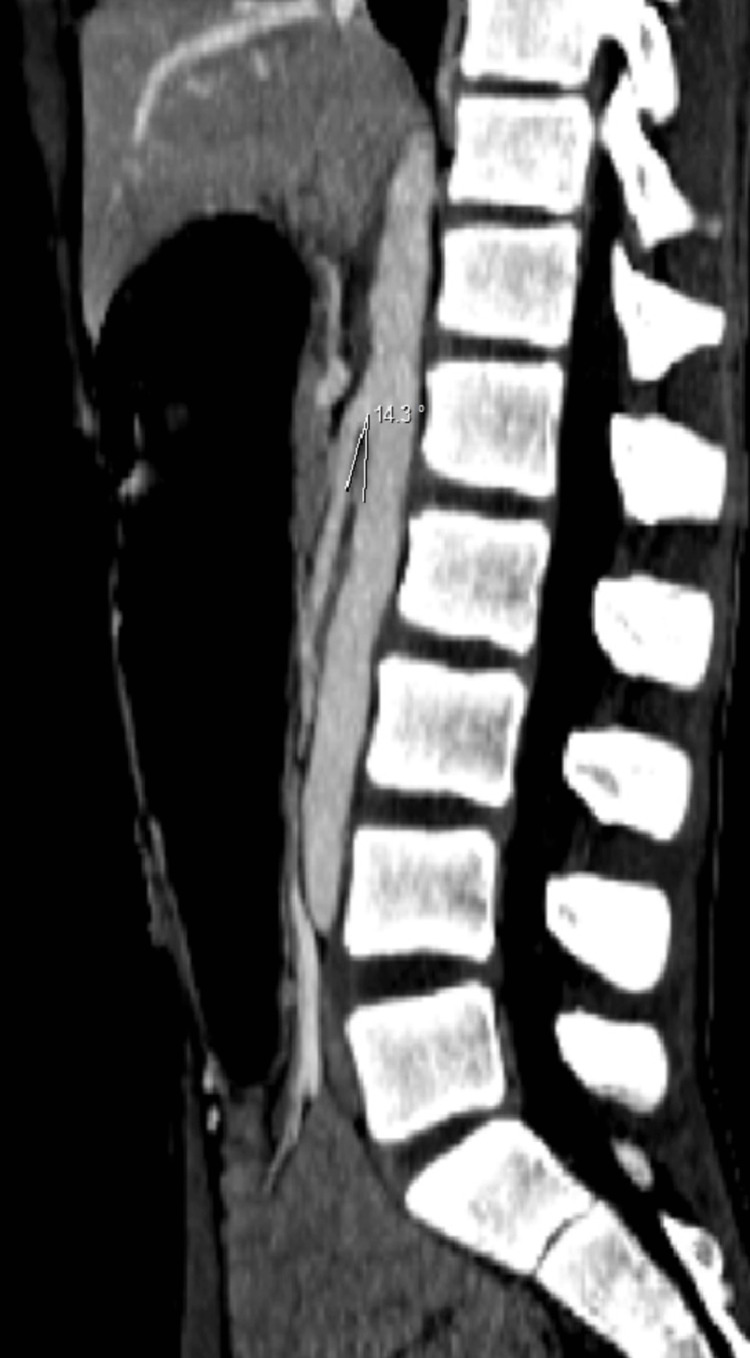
Contrast-enhanced computed tomography (CT); sagittal reconstruction of the abdomen demonstrates a reduced aortomesenteric angle measuring 14.3°.
